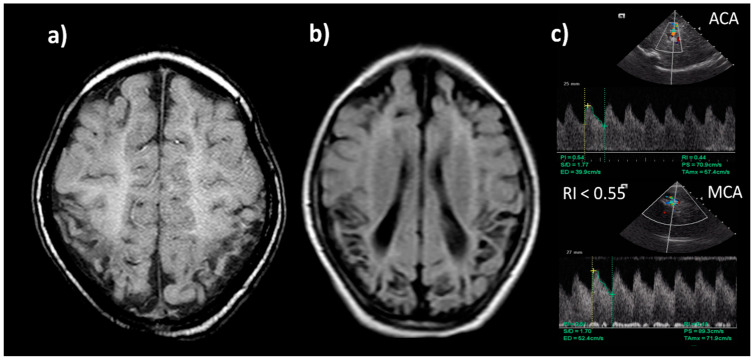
Magnetic resonance: (a) axial image of sequence enhanced in T1 towards convexity and (b) enhanced in FLAIR, where hyperintensity of the bilateral semioval centers is observed, as well as a decrease in volume in bordering territory in parietal regions and in the smaller frontal portion with retraction of the lateral ventricles associated with hyperintensity of the periventricular white matter. Courtesy: Eduardo M. Flores Armas, MD, Department of Medical Imaging. Cranial ultrasound: (c) low RI (<0.55) in normothermic infants or after rewarming has an 84% positive predictive value for death or disability. Courtesy: Daniel Ibarra-Ríos, MD, Department of Neonatology, Hospital Infantil de México Federico Gómez. ACA: anterior cerebral artery; MCA: medial cerebral artery; RI: resistive index.
